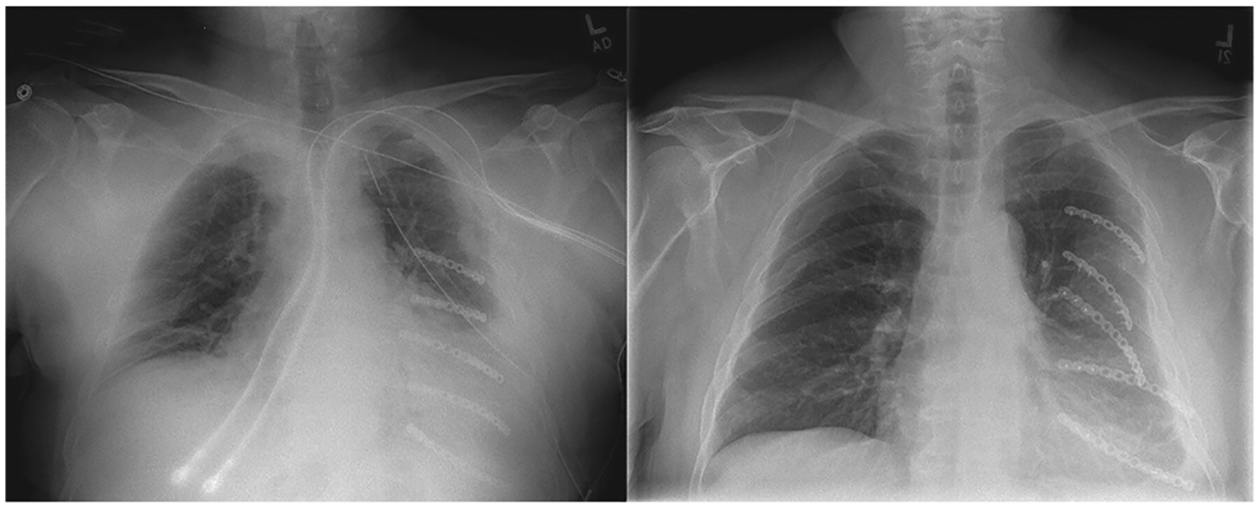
Immediate postoperative and first clinic follow-up chest X-ray demonstrating progressive hardware failure with displacement of the plates
